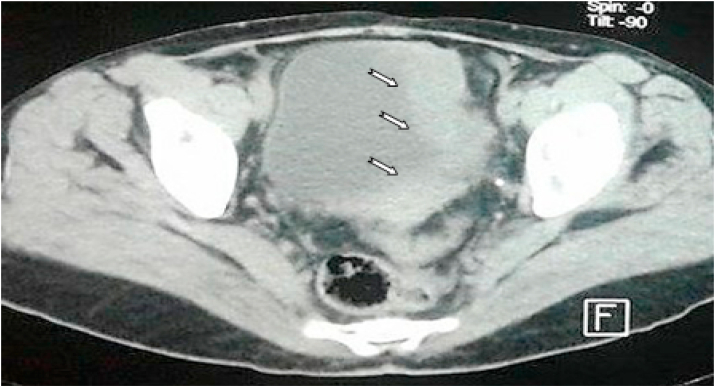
Abdomino-pelvic CT revealed bladder tumor process budding at the expense of the left lateral wall.
